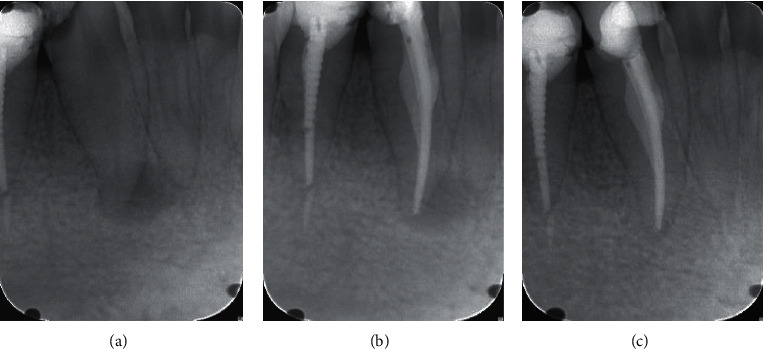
Medium-size lesion in initial treatment without extruded sealer periapical lesion. A:diagnostic X-ray; B: final obturation; C: 24 month follow-up.
